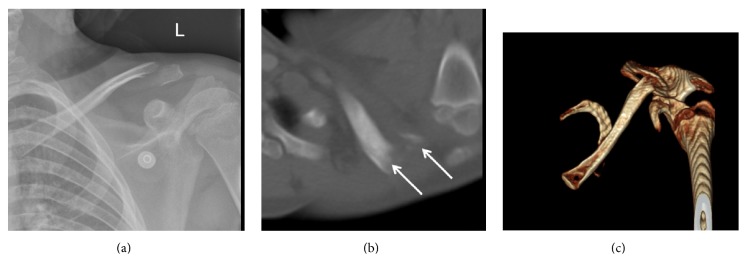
(a) Radiograph of the left shoulder showing fracture of the lateral metaphyseal clavicle. (b) CT scan of the left shoulder showing the posterior displacement of the proximal end of the fracture; arrows are pointing to the fracture ends. (c) 3D reformat of the CT of the left shoulder (view from above); notice the clavicle is posteriorly displaced in relation to the acromion and scapular spine.
